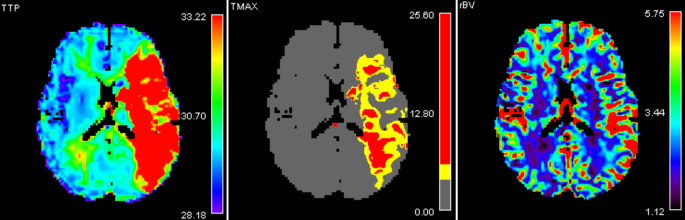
MRI Time-to-peak (TTP, right) and Tmax (middle) perfusion maps reveals a severe delay in the entire left middle cerebral artery territory with preserved cerebral blood volume (CBV, left)
